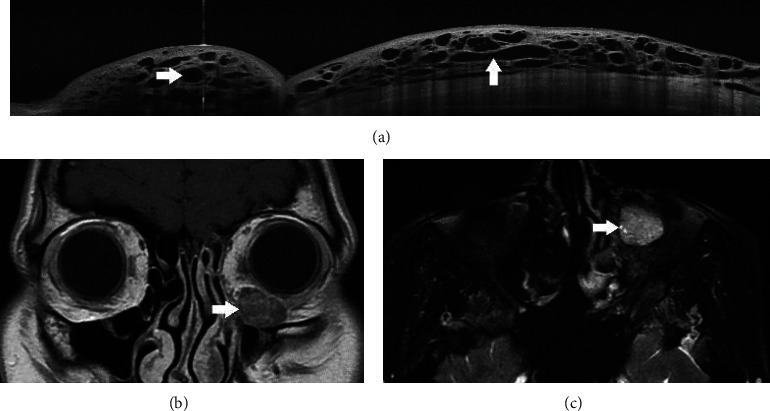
(a) Anterior segment swept source optical coherence tomography of the left eye demonstrates dilated lymphatic channels manifesting as hyporeflective spaces with different sizes demarcated by hyperreflective septae (arrows). (b) T1-weighted coronal MR image shows the inferonasally located intraconal tumor that is isointense with respect to muscle (arrow) in the left orbit. (c) T2-weighted axial MR image shows that the left orbital tumor is hyperintense (arrow).
